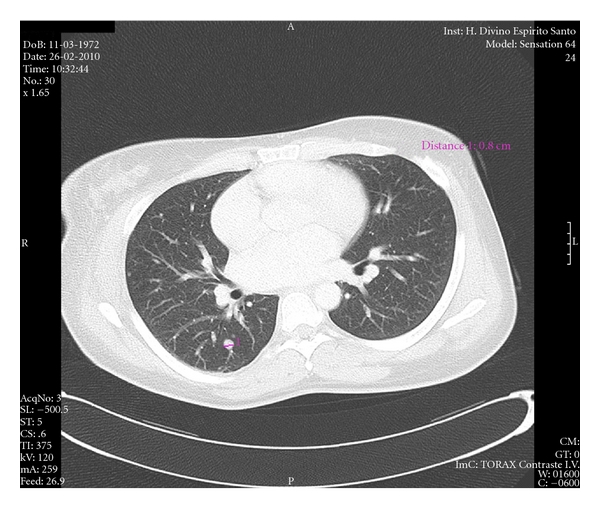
Thoracic CT scan. Multiple micronodules are visualized in peripheral topography, more numerous at the right lung, where an 8 mm nodule can be identified in the apical segment of the inferior lobe, with topography and morphology suggestive of metastases.
